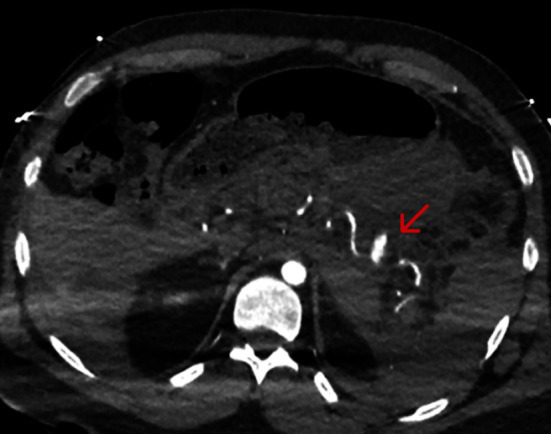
Splenic artery aneurysm with active contrast blush on abdominal CT angiogram.
